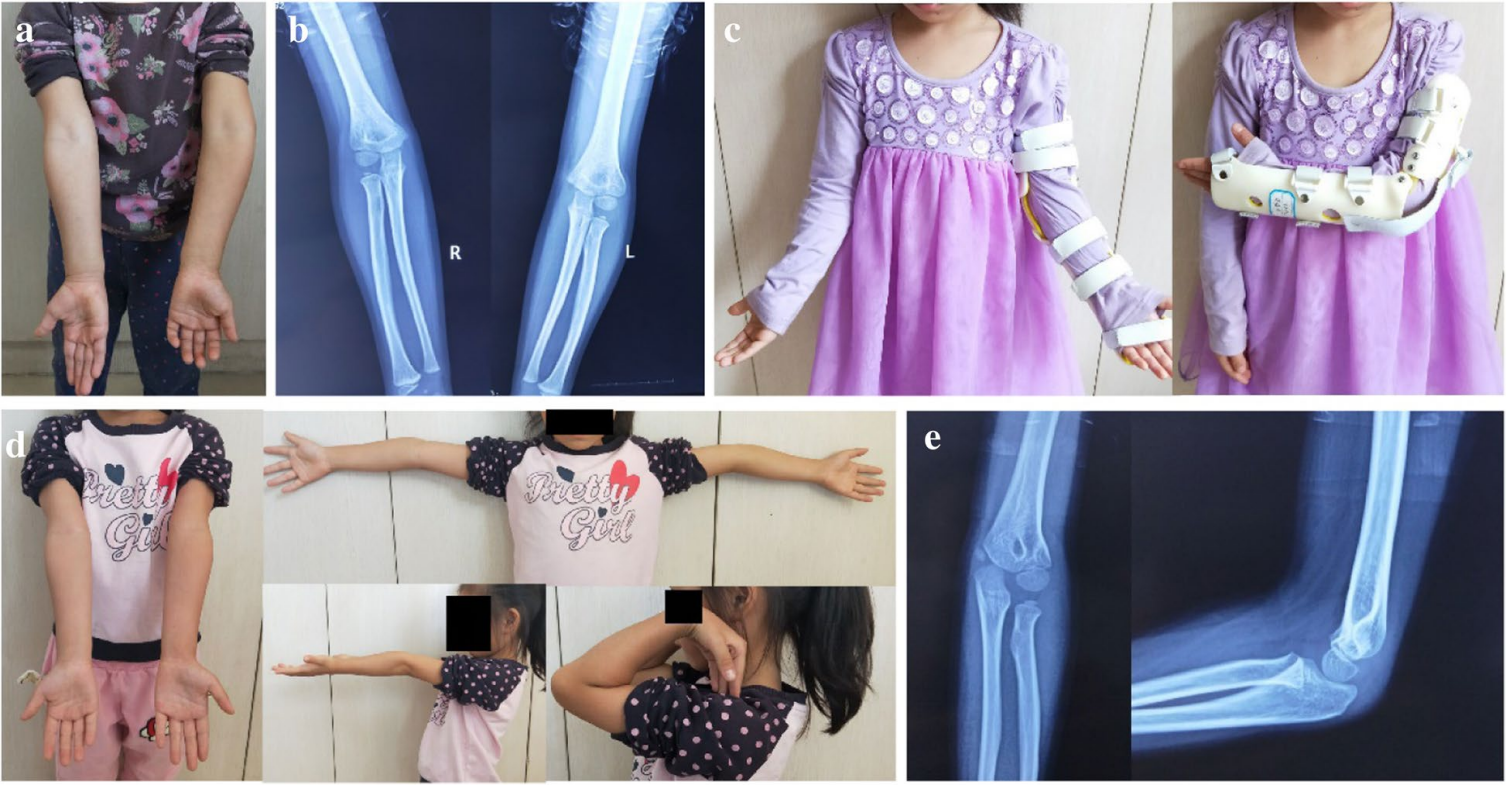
Case 1: a Pre-operative clinical appearance of bilateral upper limb (left side cubitus varus deformity). b Pre-treatment imaging. c Patients with cubitus varus wearing the functional brace. d Clinical appearances of bilateral upper limb. e Post-treatment radiographs of bilateral upper limb at last follow up
